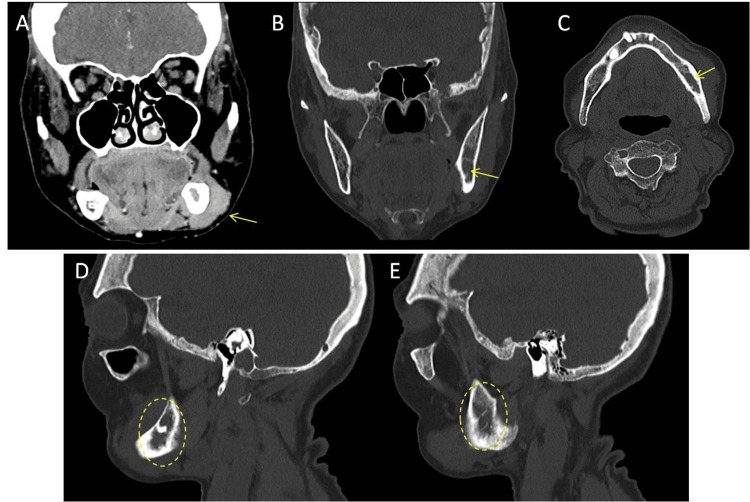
Maxillofacial CT showing an infiltrative lesion of the left mandible, consistent with extranodal follicular lymphoma. The lesion measures 40 × 30 × 30 mm (transverse × craniocaudal × anteroposterior) (A) Coronal soft tissue window demonstrating an expansive, infiltrative mass in the left buccal space (yellow arrow), extending into the mental foramen. (B) Coronal bone window showing partial erosion of the cortical bone and involvement of the inferior alveolar canal (yellow arrow). (C) Axial bone window view revealing lateral cortical disruption and inferior alveolar canal involvement (yellow arrow). (D, E) Sagittal bone window views depicting cortical thickening (suggestive of hyperostosis) and diffuse changes in the trabecular bone of the left hemimandible (dashed yellow circles), with no evident osteolytic lesions.
